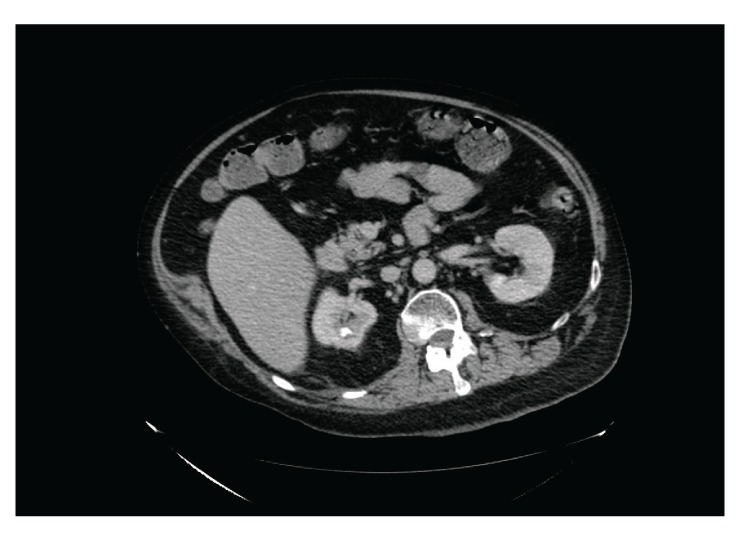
Axial section of computed tomography of abdomen and pelvis revealed 10 mm calculus in upper pole of right kidney.
